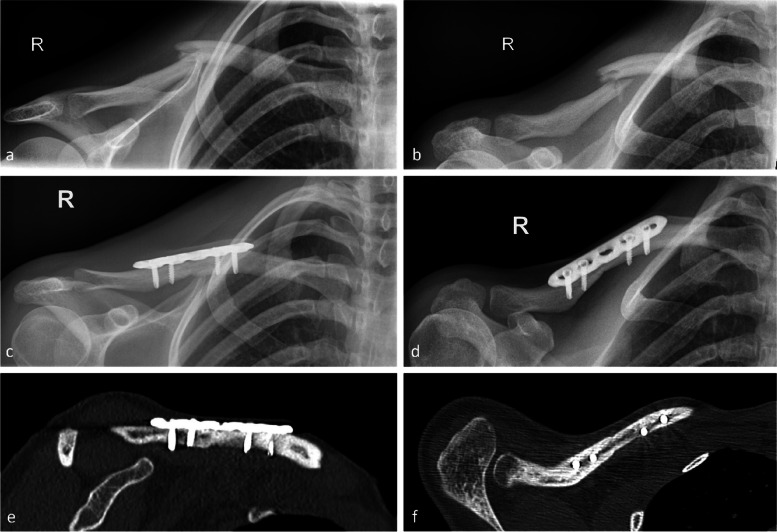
Radiological example of a 2-part clavicle shaft fracture (15.2A according to AO classification) (a and b) and radiological result 3 years (c and d) after surgical treatment using a 5-hole LCP. e and f CT scan showing a full consolidation 21 months after surgery
